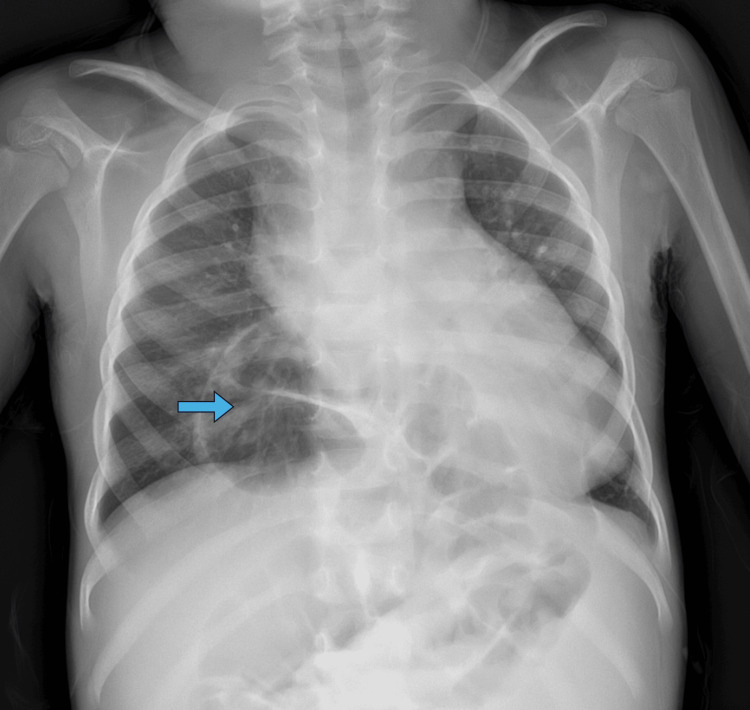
Chest radiography demonstrating herniation of the colon into the thorax (blue arrow).
